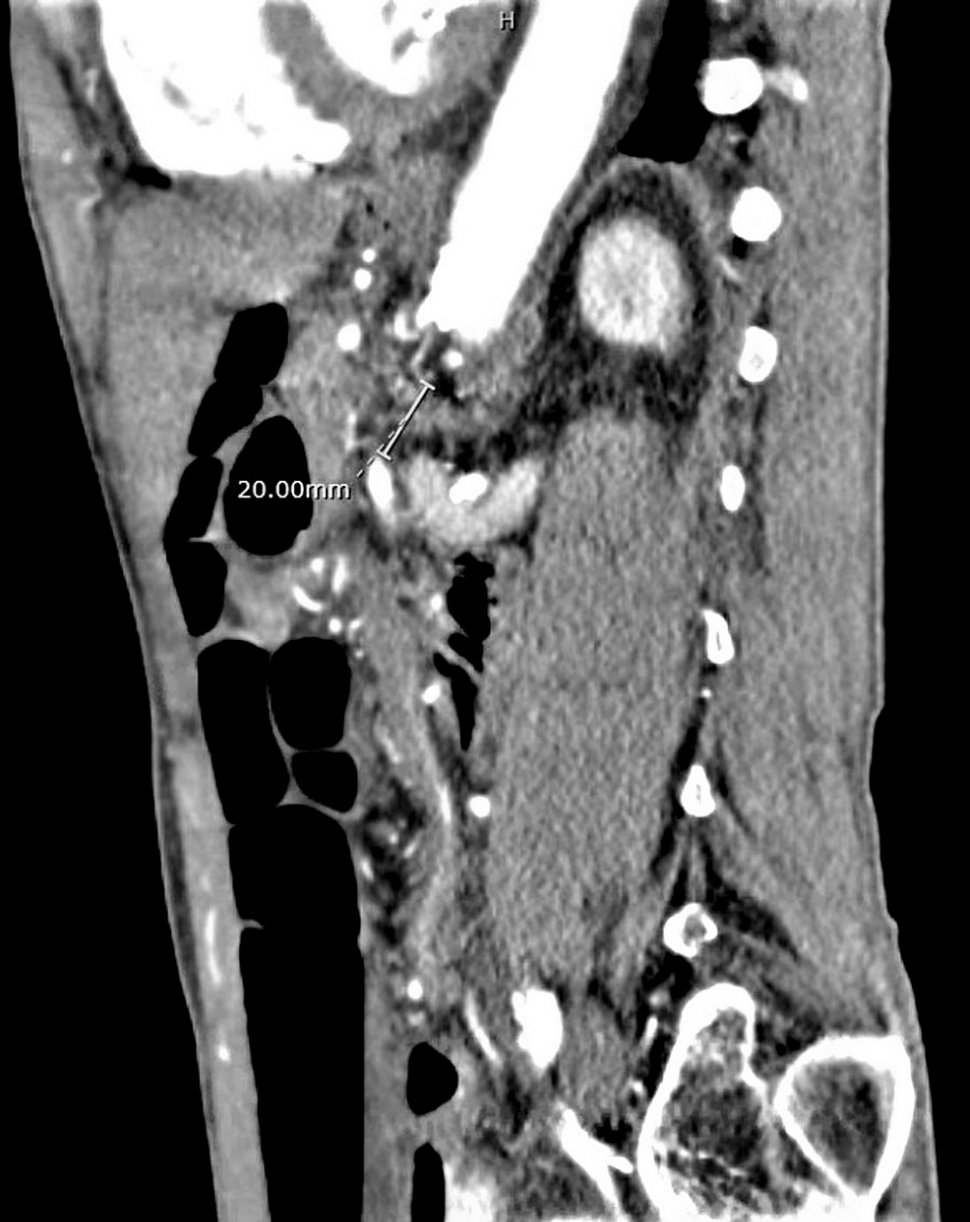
CT Angiography showing a 20 mm occlusion at the origin of the superior mesenteric artery
